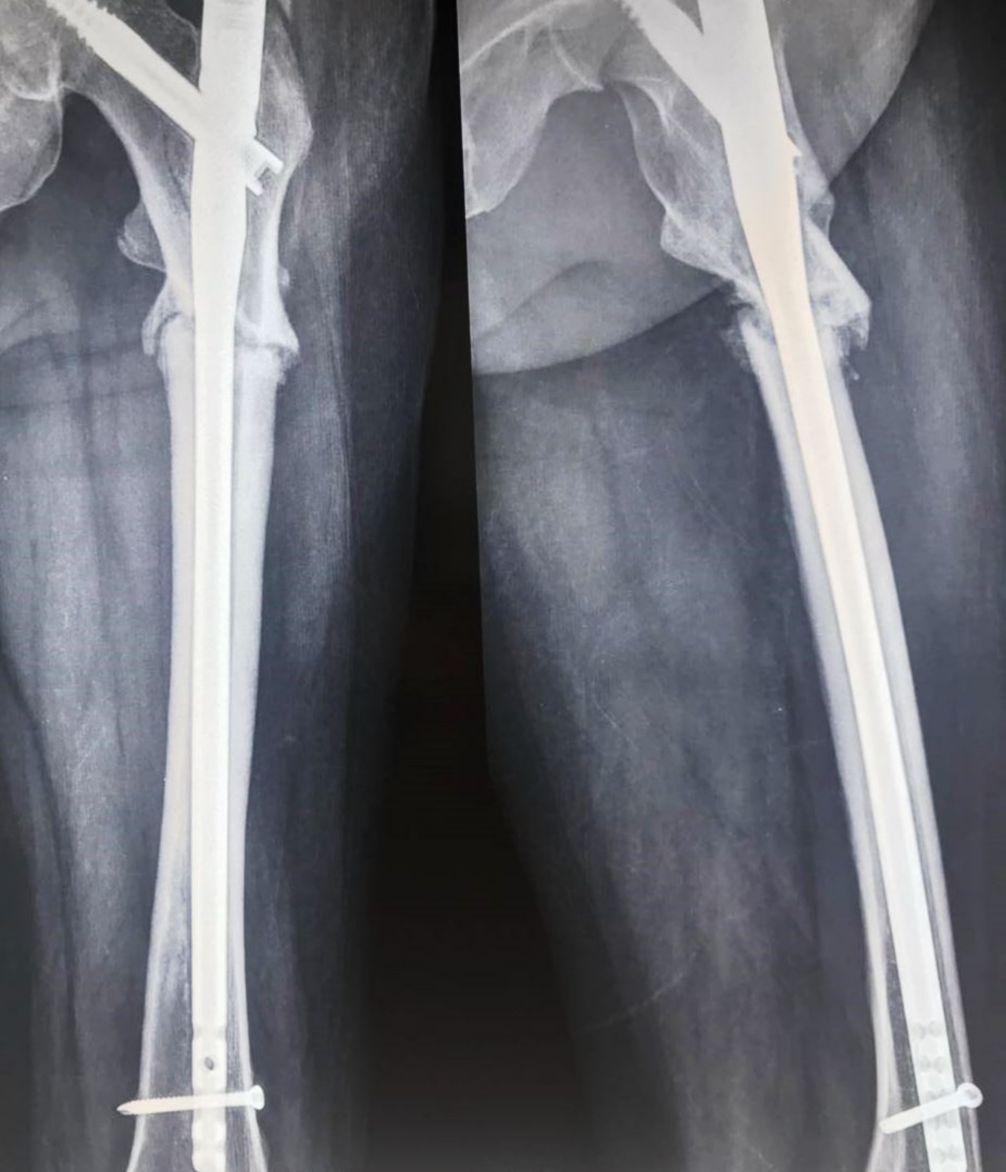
One-year postoperative X-ray showing nonunion at the fracture site.
